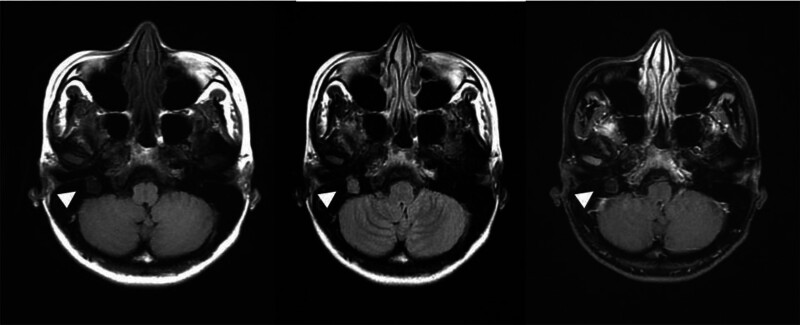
Lesion in right middle ear cavity: hypointense in T1-weighted scan (left), intermediate to hyperintense in T2-weighted scan (middle), and exhibited ring enhancement in postcontrast T1 scan (right).
